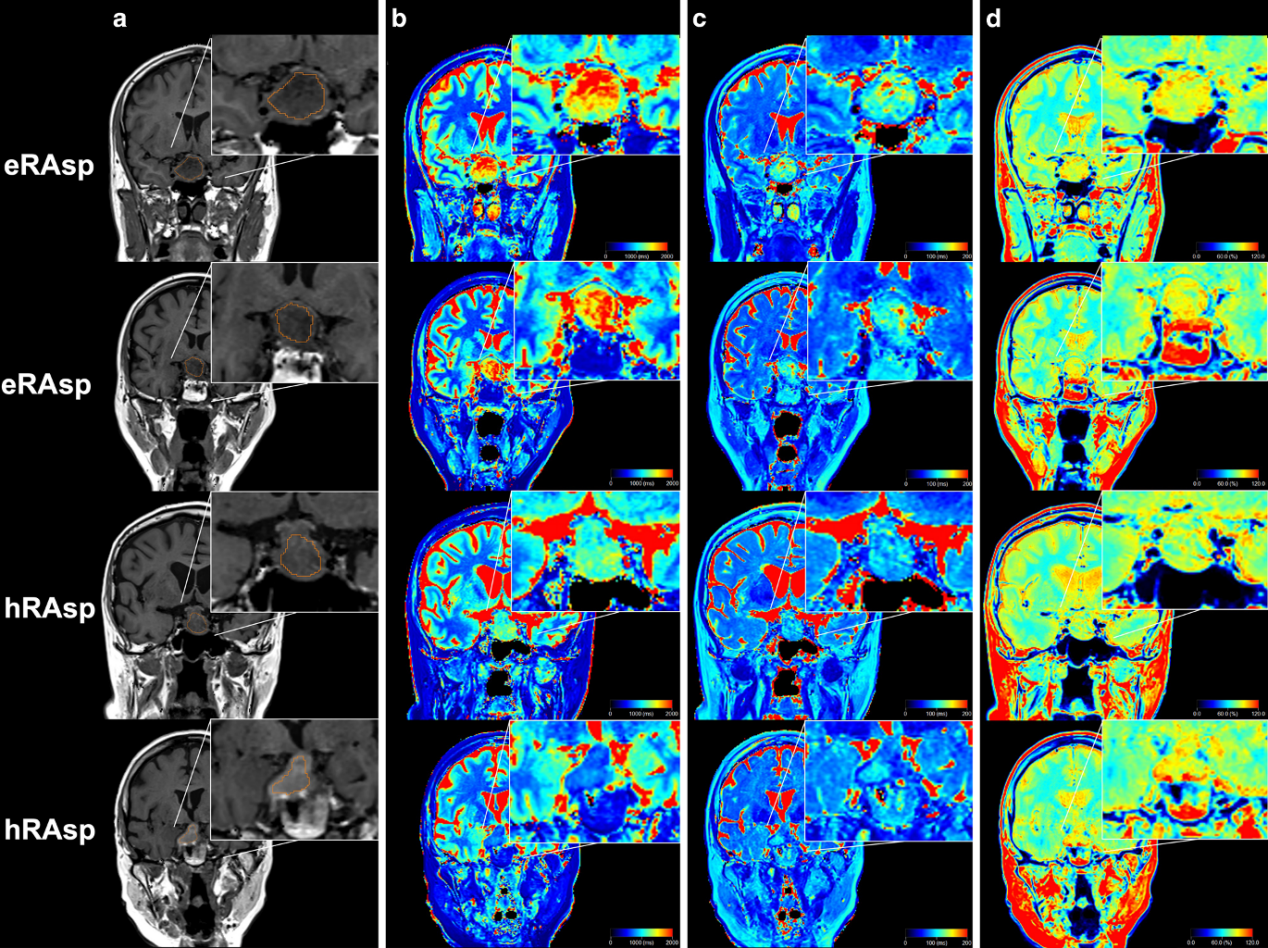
Non-enhanced, multi-dynamic-multi-echo (MDME)-based T1-weighted contrasts (a); quantitative T1-maps (b); quantitative T2-maps (c); and quantitative proton density (PD)-maps (d)are demonstrated (coronal plane). MR data of four different patients (*rows*) are presented: *1st row*, male subject, 34 years of age at the time of data acquisition (gonadotroph adenoma) [physical MR metrics based on the measurements of rater 1: 1741 ms (T1-relaxation time), 120 ms (T2-relaxation time), 85.3% (proton density)]; *2nd row*, female subject, 55 years of age at the time of data acquisition (null cell adenoma) [physical MR metrics based on the measurements of rater 1: 1709 ms (T1-relaxation time), 116 ms (T2-relaxation time), 85.7% (proton density)]; *3rd row*, male subject, 76 years of age at the time of data acquisition (null cell adenoma) [physical MR metrics based on the measurements of rater 1: 1165 ms (T1-relaxation time), 89 ms (T2-relaxation time), 80.5% (proton density)]; *4th row*, female subject, 65 years of age at the time of data acquisition (null cell adenoma) [physical MR metrics based on the measurements of rater 1: 865 ms (T1-relaxation time), 85 ms (T2-relaxation time), 71.8% (proton density)]. Region of interest (ROI) placement is demonstrated on the MDME-based T1-weighted contrasts (a). The colored bars indicate the T1-relaxation times [ms], T2-relaxation times [ms], and proton densities [%]. The relaxometric differences between “hard-to-remove by aspiration” (hRAsp) vs. “easy-to-remove by aspiration” (eRAsp) adenomas are well appreciated on the quantitative maps
